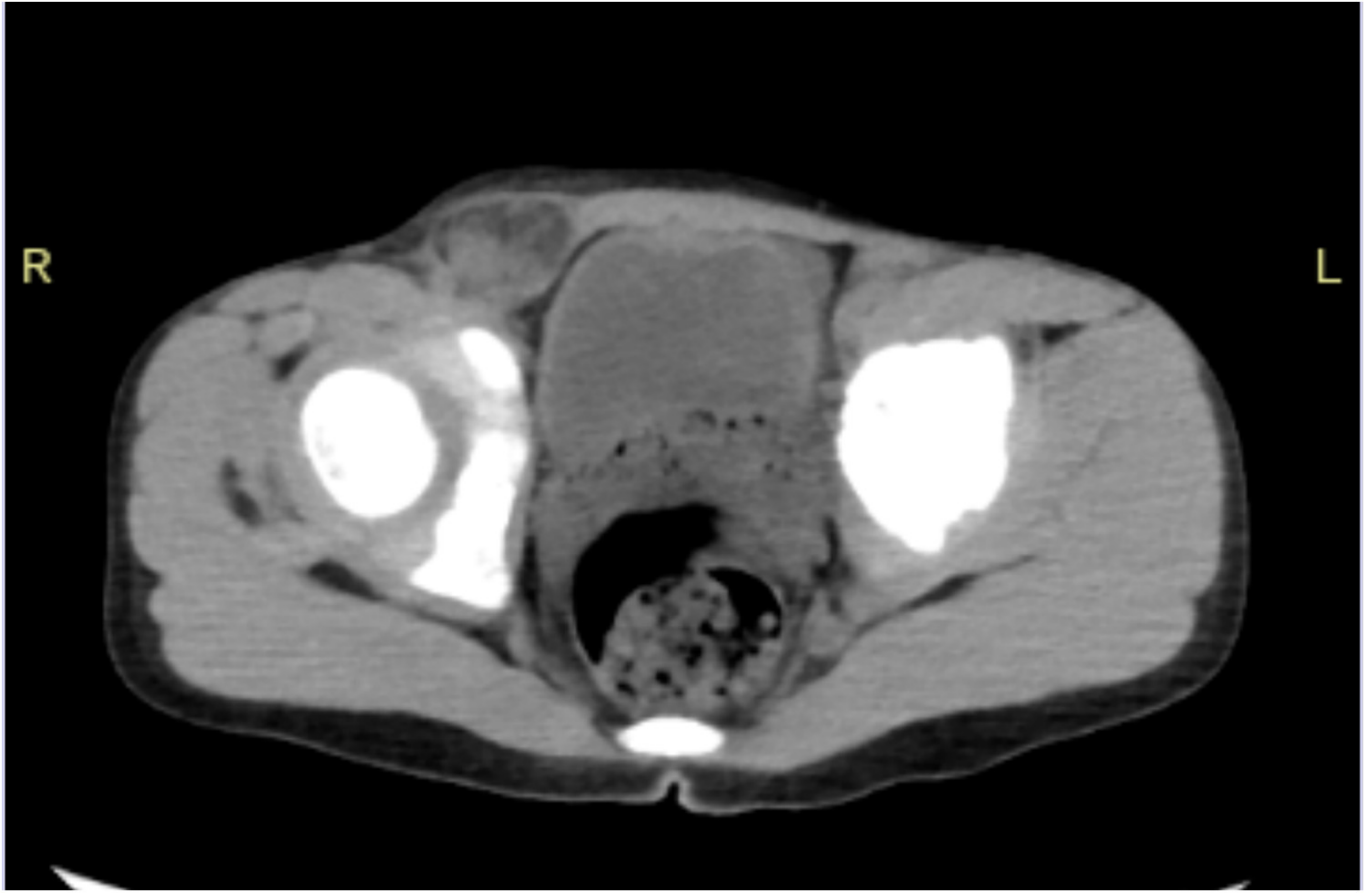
CT of the lower abdomen showed a part of the small intestine and fat in the right inguinal region herniated into the right hernial sac.
